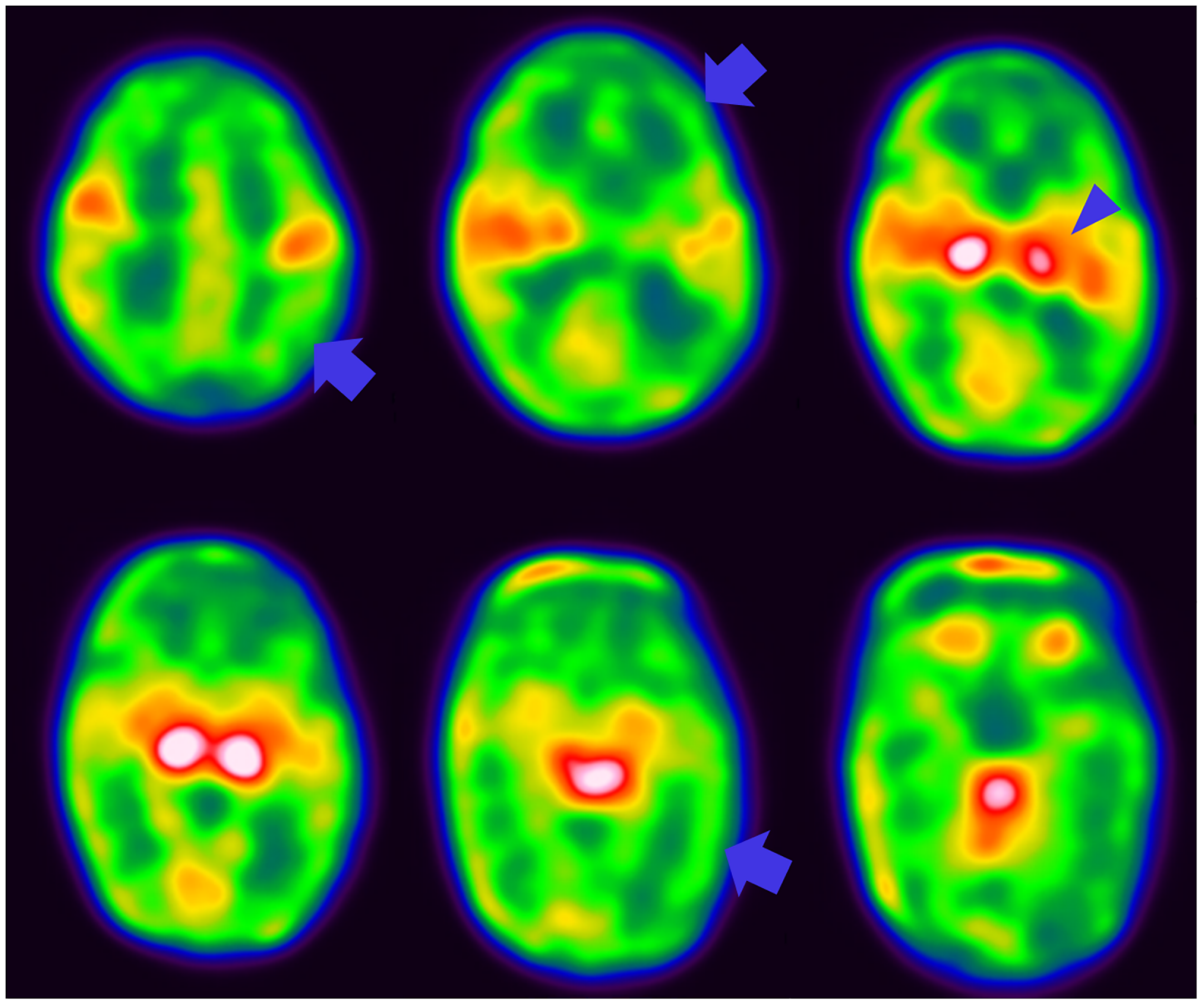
A representative F-18 FDG brain PET image from a very low-birth-weight infant. It shows relatively low glucose metabolism in the right cerebral cortex (arrow) and left thalamus (arrow head) relative to the corresponding regions in the left hemisphere.
